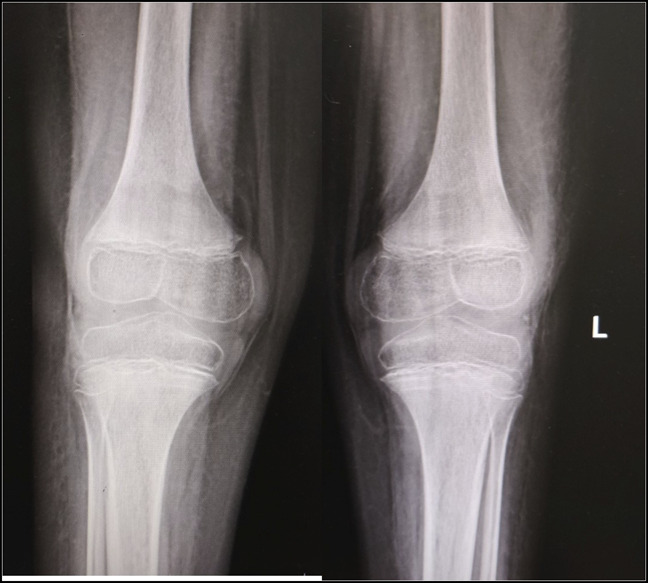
Radiograph of AP of both knees showing osteopenia, ring sign (Wimberger) in epiphysis, radiodense (Frenkel), and lucent (Trummerfeld) lines along the metaphysis and metaphyseal (Pelkan) spurs.
